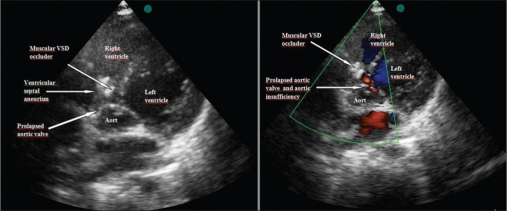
Closing of VSD using Cardi-O-Fix muscular VSD occluder and checking of the aortic insufficiency shortly after releasing the device on transthoracic echocardiographic imaging
